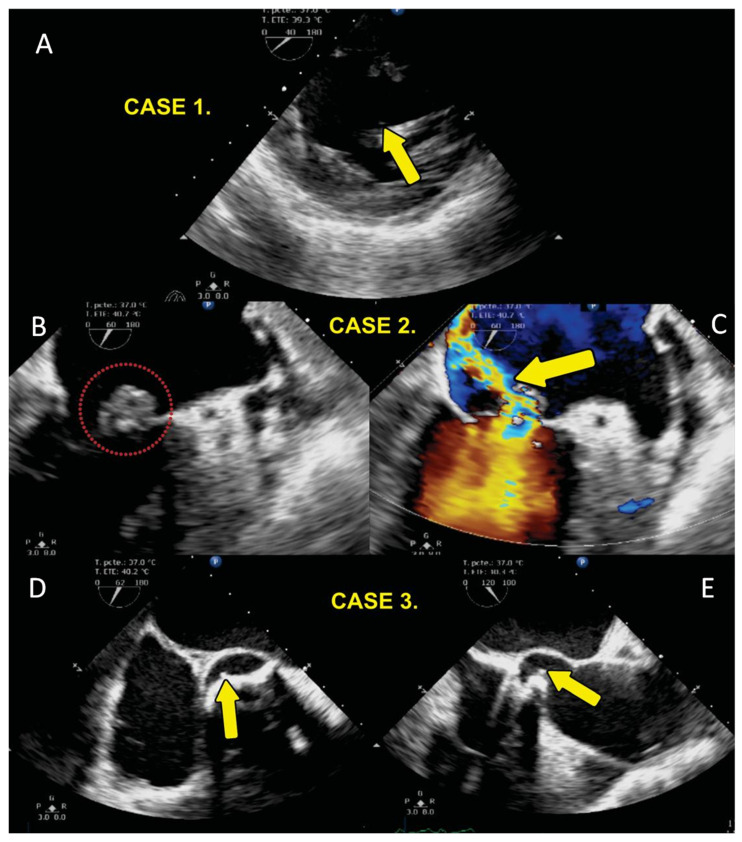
Transesophageal echocardiographic analysis of the patient series. Panel (A): case 1. Transgastric short axis showing vegetation (yellow arrow) in relation to the subvalvular mitral apparatus. Case 2 showing mitral valve endocarditis (red circle) in panel (B) and severe mitral regurgitation (yellow arrow) in panel (C). Case 3. Transesophageal short axis in panel (D) and three-chamber view in panel (E), showing vegetation in the aortic valve (yellow arrow).
